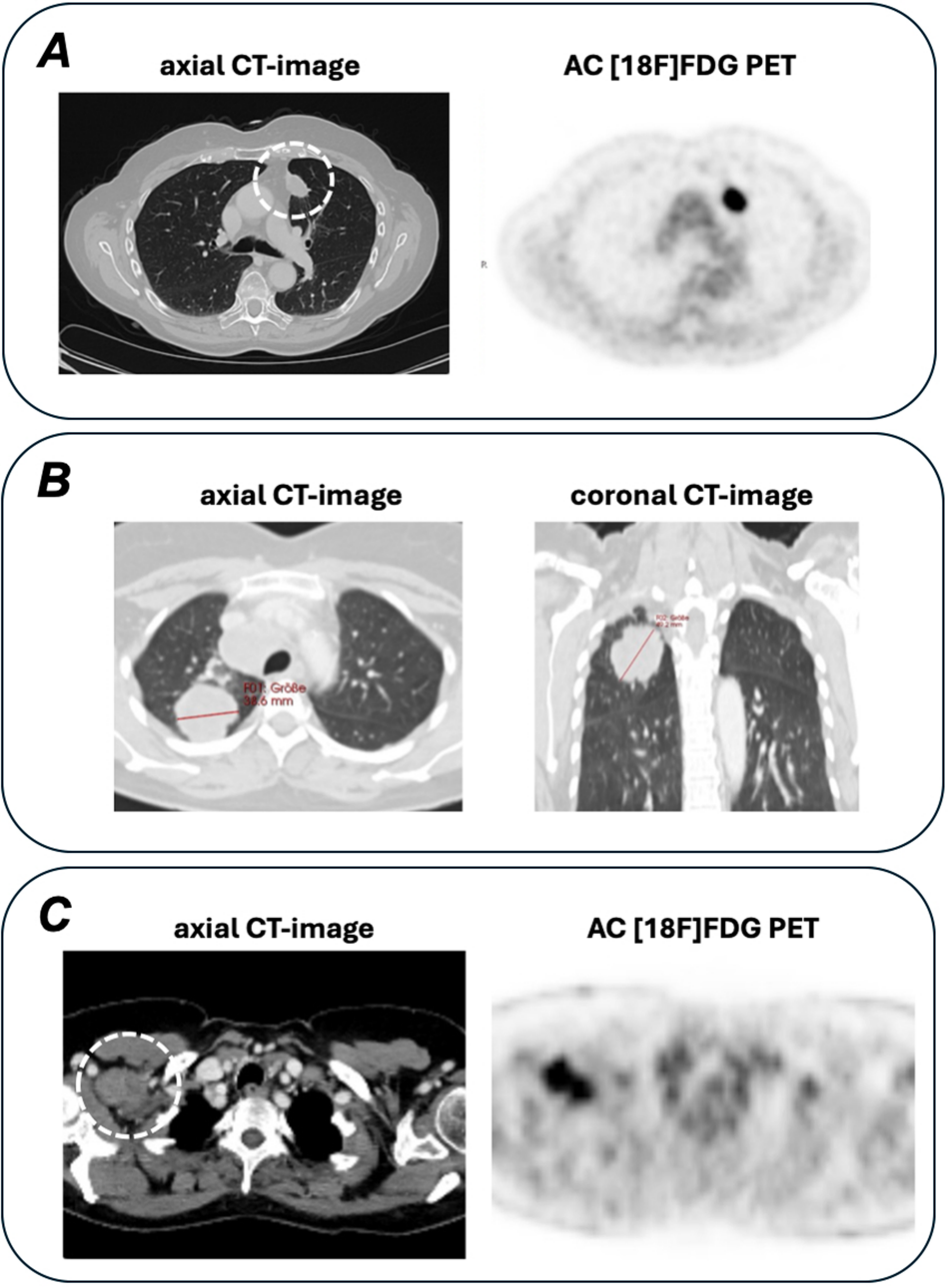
Representative classification errors in SR and FTR. A Shows the primary tumor in patient 3 with broad visceral pleural contact indicative of an infiltration of the visceral pleura (T2a), as compared to size-based stage T1c. B Shows the primary tumor in patient 13 demonstrating a maximum multiplanar diameter in the coronal plane of 4.9 cm (T2b) as compared to the maximum axial diameter of 3.9 cm (T2a). C Shows a right-sided axillary lymph node metastasis consistent with an extrathoracic metastasis instead of a regional lymph node (N3) as it is not included in the International Association for the Study of Lung Cancer (IASLC) map. SR, structured reporting; FTR, free text reporting; AC, attenuation correction; FDG, fluorodeoxyglucose
